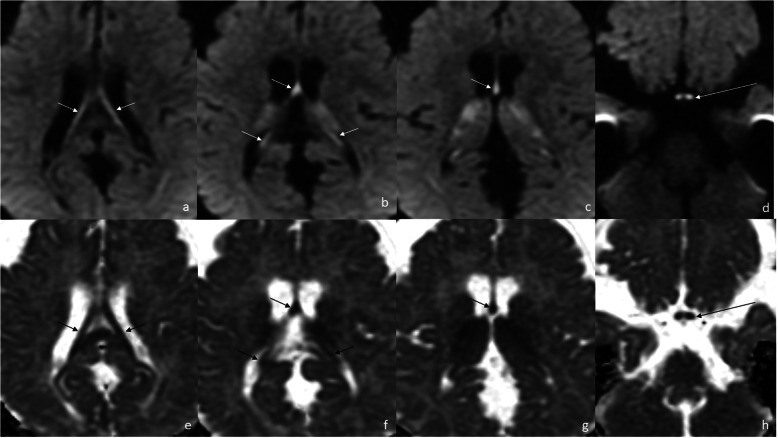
Scan 1) at the age of 8 months. Diffusion restriction in the fornix (a-c, e-g, short arrows) and in the optic chiasm (d, h) (a-d: DWI, e-h: ADC maps, long arrows). The involvement of the globi pallidi is also seen (c, g)
